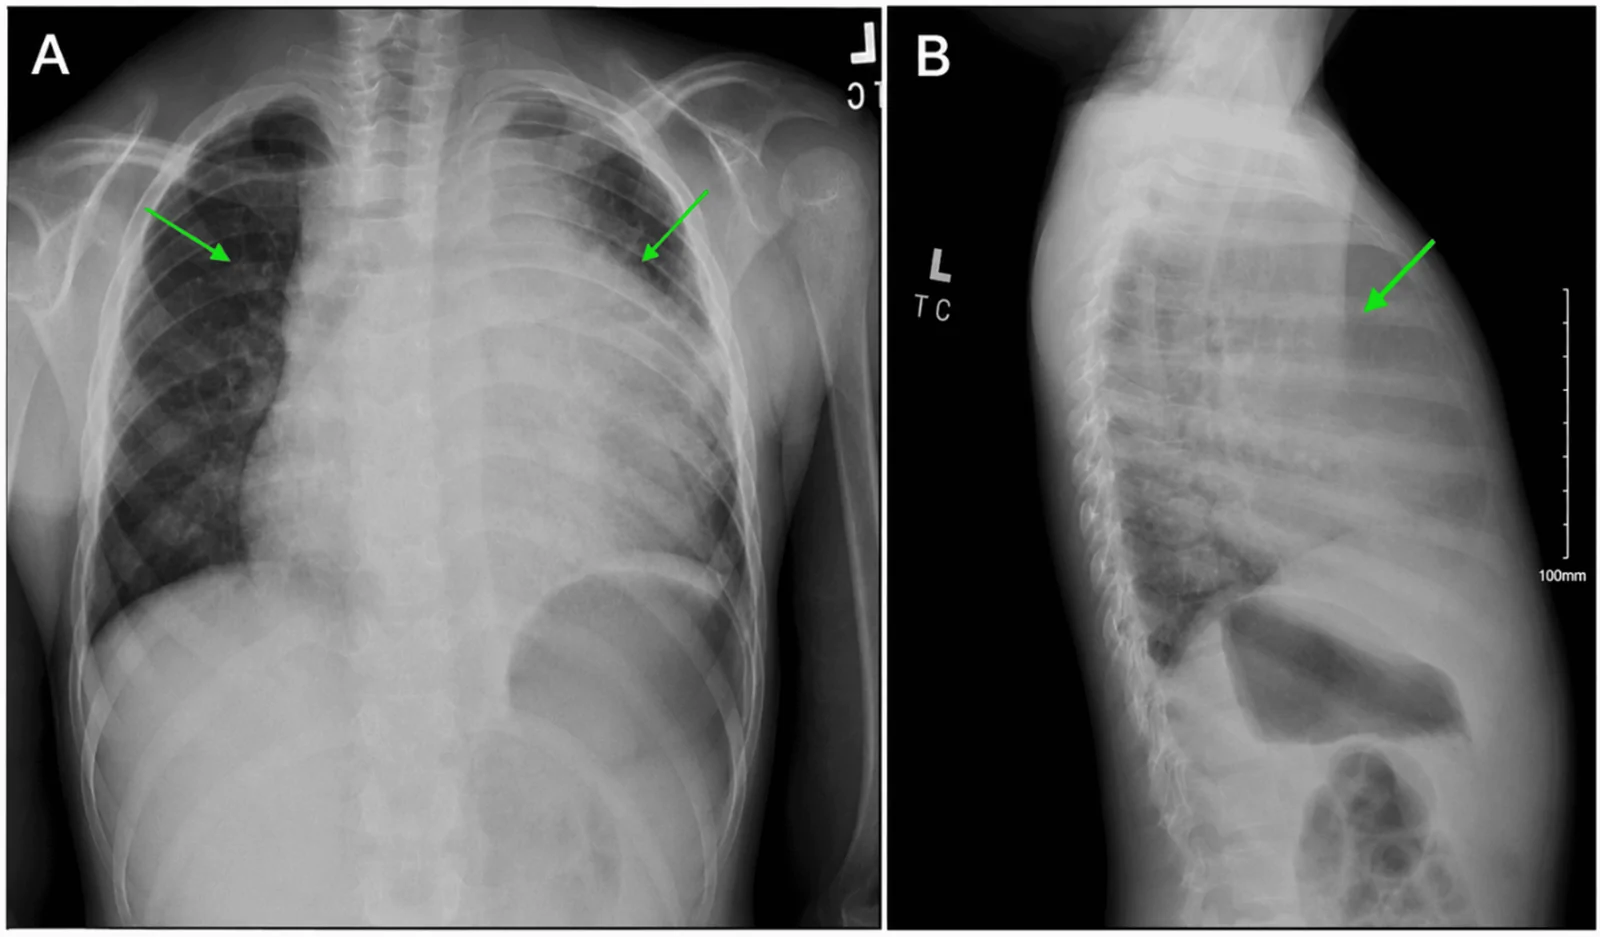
Chest X-ray (A) PA view and (B) lateral view showing mediastinal widening (green arrows) and cardiomegaly.
